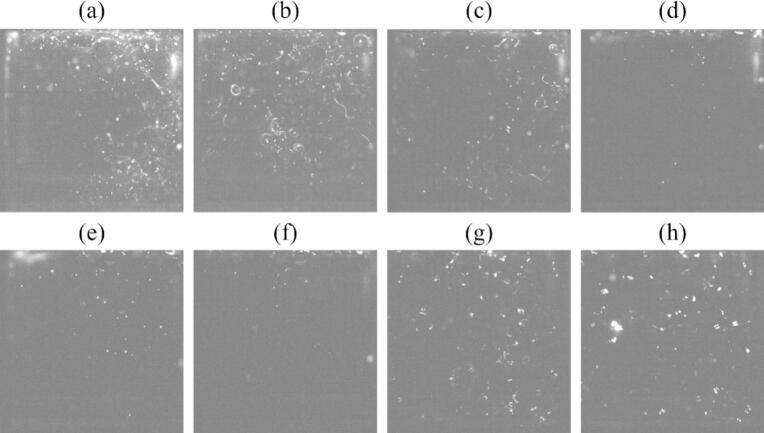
Snapshots of bubbles visualized by the green laser sheet in the ultrasonic bath with the ultrasonic power of (a) 18 W, (b) 33 W, (c) 43 W, (d) 49 W, (e) 69 W, (f) 84 W, (g) 117 W, and (h) 136 W.
